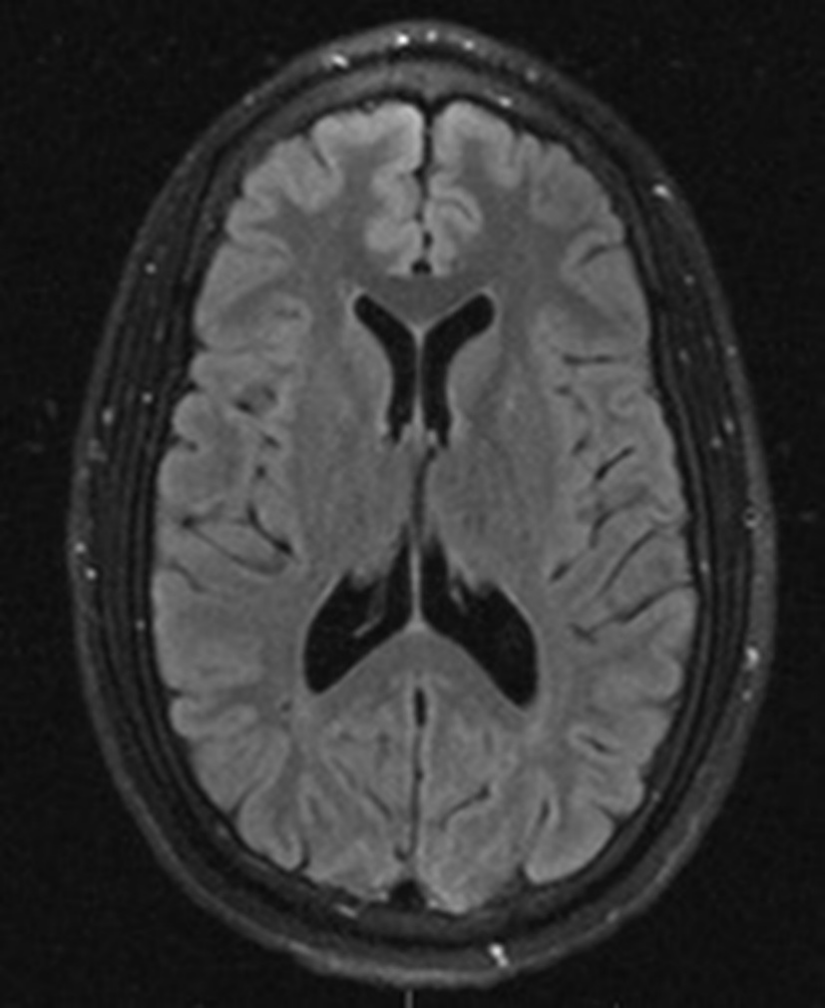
Axial view T2 FLAIR MRI imaging 2 years after initial presentation showed complete resolution of brain lesions.
